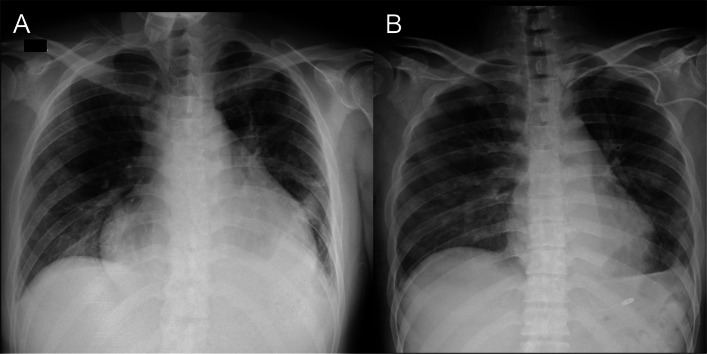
Chest radiography. A Chest radiography on the day of respiratory deterioration, demonstrating marked cardiomegaly. B Chest radiography on the day after decannulation from ECMO and removal of mechanical ventilation, revealing improvement in the cardiomegaly
